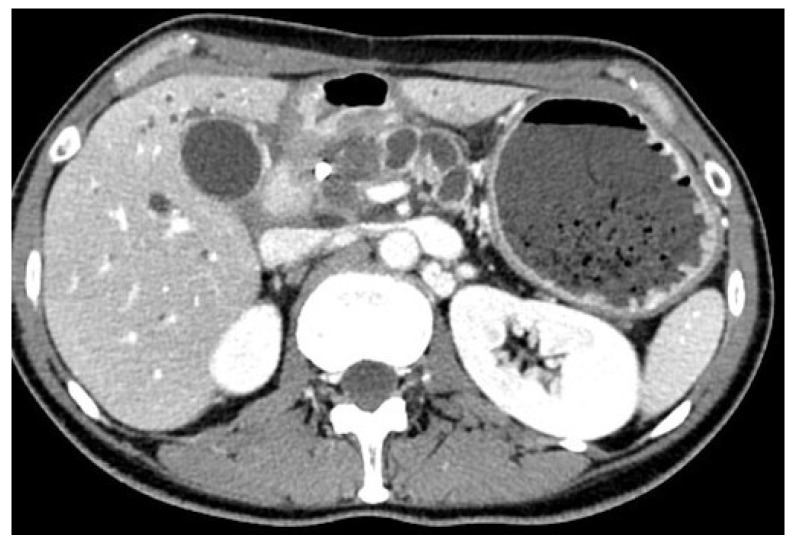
Computed tomography scan: a case of concomitant biliary and duodenal obstruction. From the personal archive of the corresponding author.
